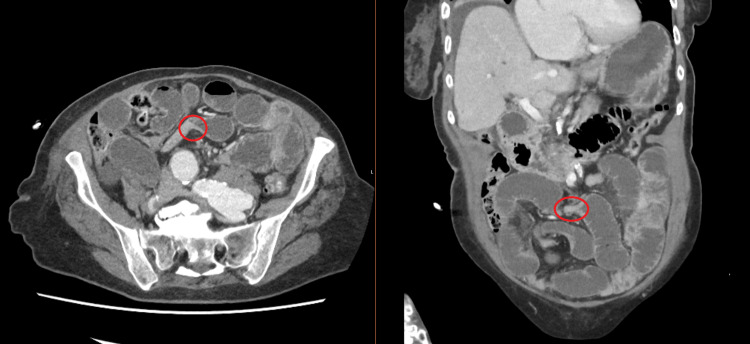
Axial and coronal portal venous phase CT images demonstrating a high-grade small bowel obstruction, with a transition point noted in the right iliac fossa
